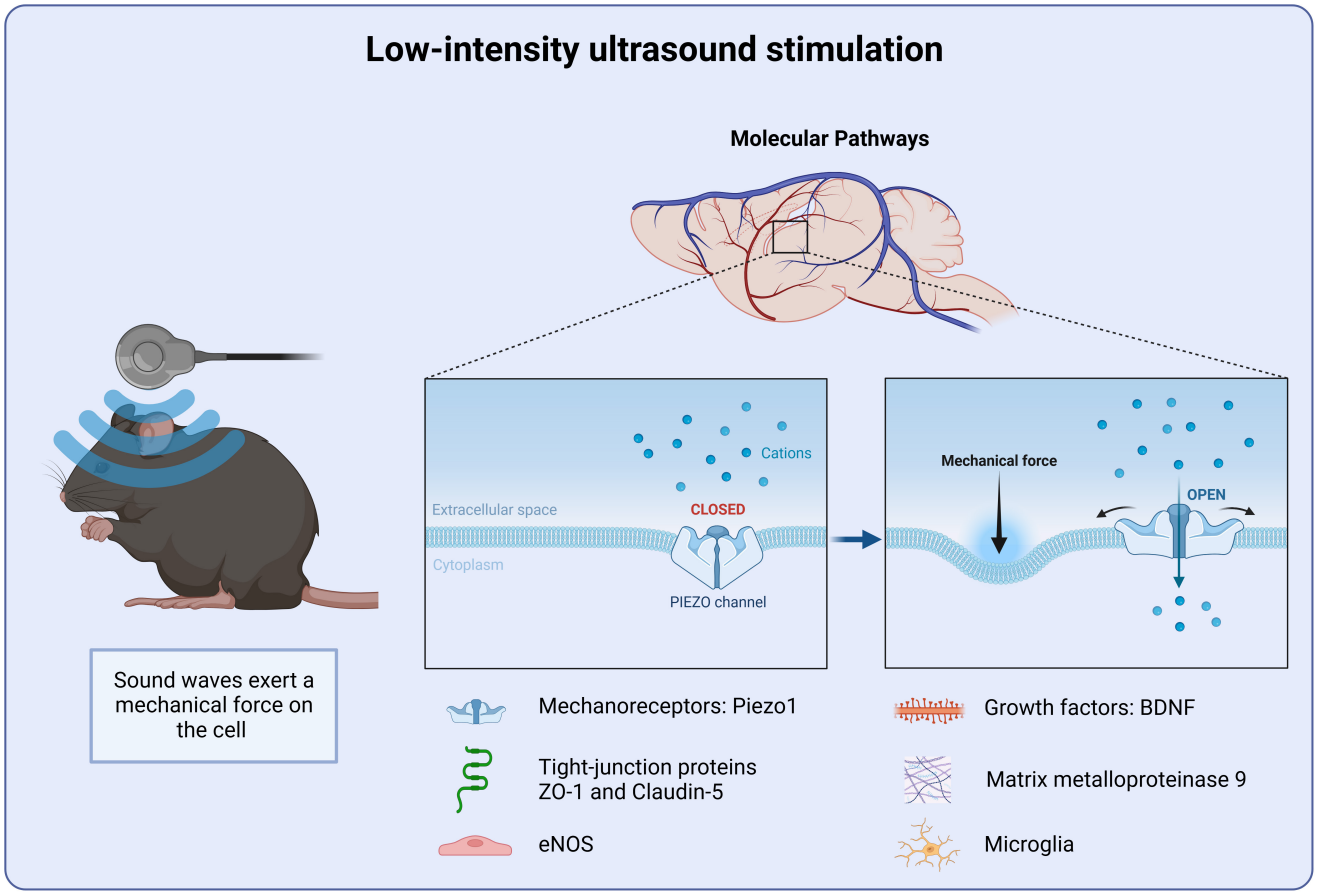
Molecular targets of Low-intensity ultrasound stimulation. Low intensity ultrasound stimulation targets the mechanoreceptor Piezo1. Piezo1 detects changes in the mechanical force of the cellular microenvironment. Piezo1 helps to regulate intracellular calcium homeostasis and preventing further inflammatory signal cascade.
